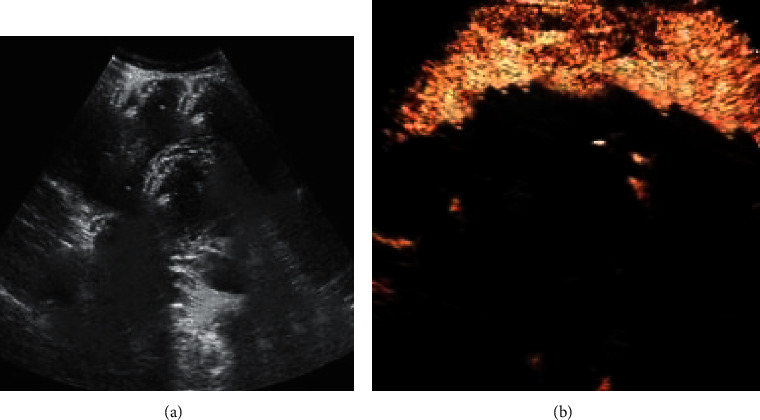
Contrast ultrasound image: (a) two-dimensional ultrasound image; (b) contrast-enhanced ultrasound.
